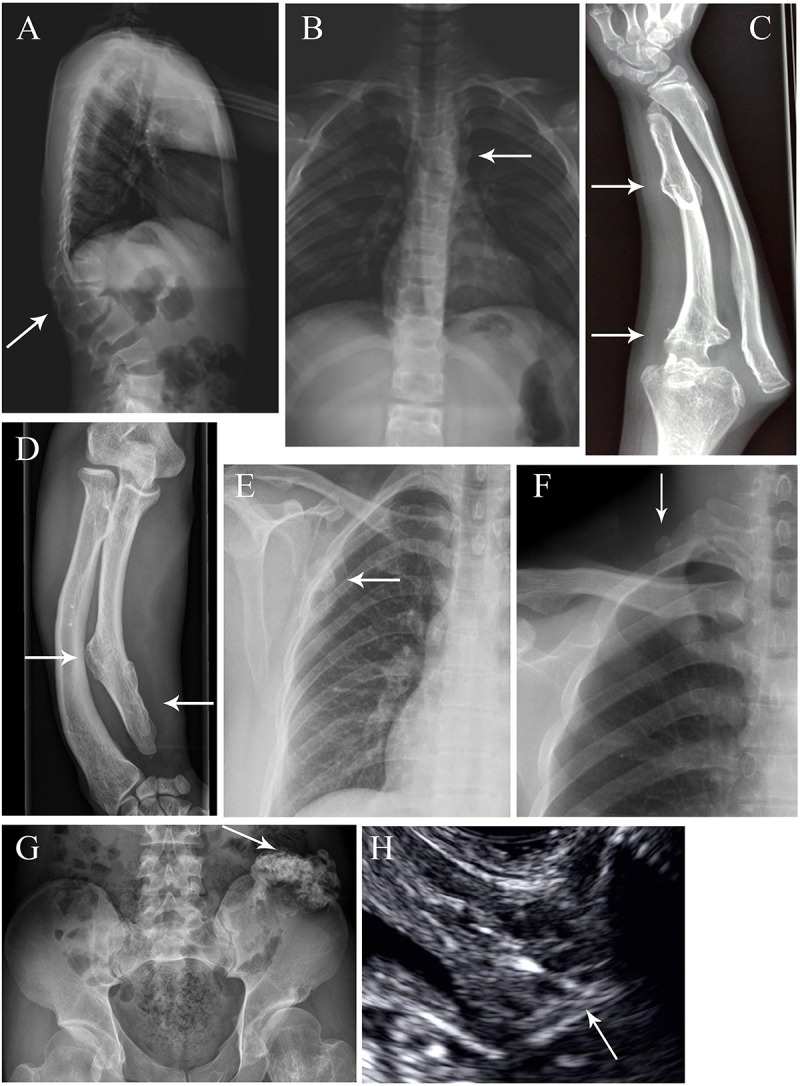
Special exostosis lesions and complications in some affected MO individuals. (A,B) Radiographs of Family 13 member III-5 as anteroposterior and lateral views, revealing the scoliosis, thoracolumbar kyphosis and vertebral fusion (arrow). (C) Right forearm of Family 11 member III-1, showing exostosis in the ulna and dislocation of the elbow joint (arrow). (D) Right forearm of Family 4 member II-2, showing exostosis in the ulna and bowed forearm conferring dislocation of radioulnar joint (arrow). (E) Radiograph of Family 7 member III-1, revealing the exostoses in the scapula (arrow). (F) Radiograph of Family 4 member II-2, showing the exostosis at the right side of the first rib (arrow). (G) Pelvic radiograph of Family 4 member II-2, displaying exostosis on the left side of the ilium (arrow). (H) Ultrasound images of Family 7 member III-1, showing the absence of a uterus (arrow).
